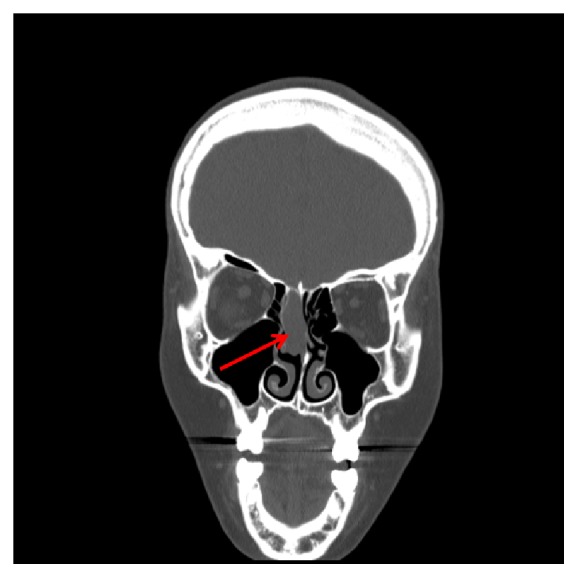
CT sinuses (coronal) for Case 2. There is a 3 cm ovoid opacity in the mid to superior aspect of the right nasal cavity with extension to the roof of the nasal cavity.
